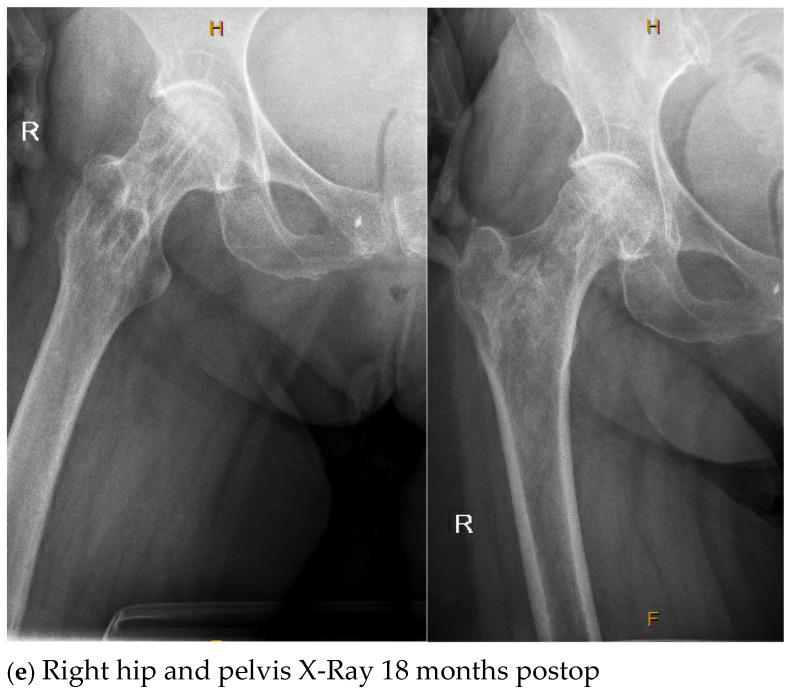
X-Ray images depicting surgical management (Case 4).
